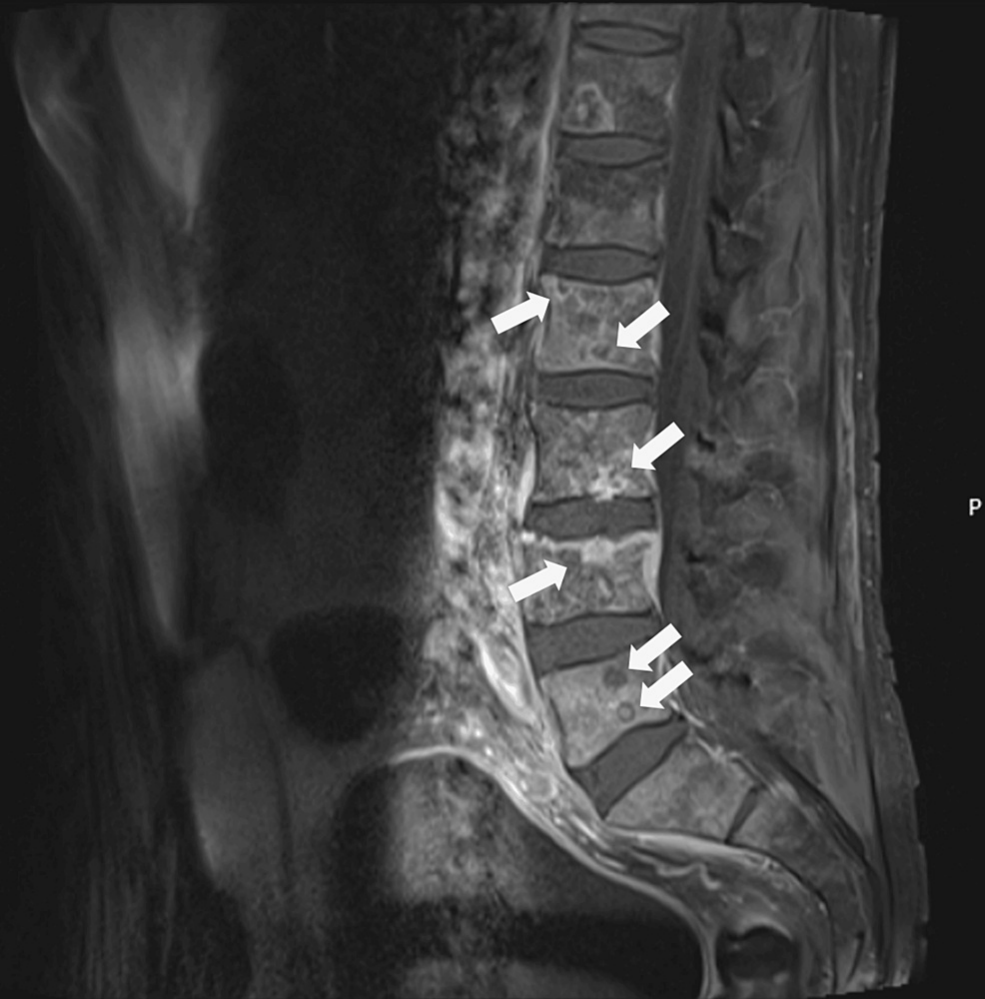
MRI section of the lumbosacral spine with foci of metastization (white arrows)
